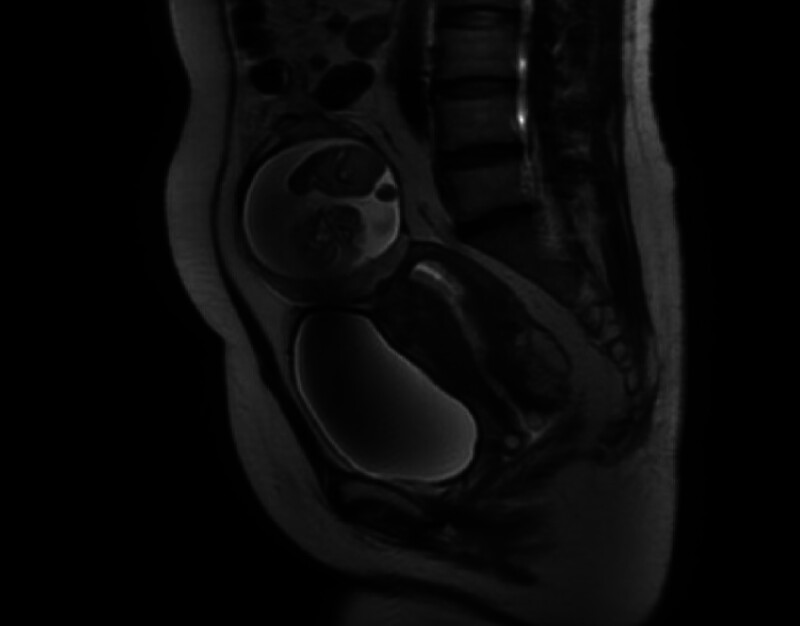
Abdominal pregnancy MRI image. MRI = magnetic resonance imaging.
